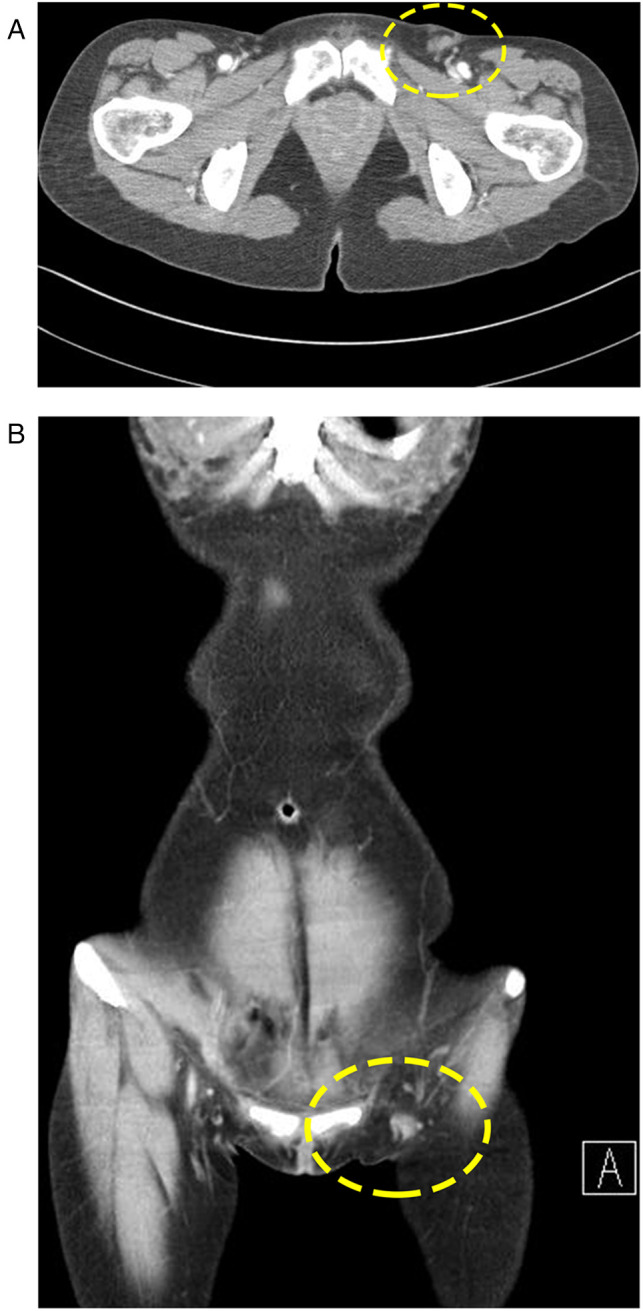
Preoperative CT scan: (A) A 1.7-cm, regional conglomeration of dense, soft tissue is evident. (B) Lesion in (A) is surrounded by benign-looking adipose tissue.
